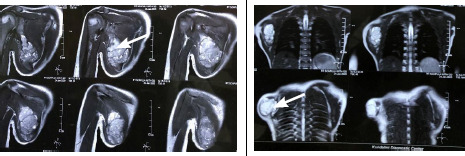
A) An MRI scan showed an ill-defined altered signal intensity lesion measuring 49x35 mm noted at the infraspinatus portion of the body of the scapula, B) heterogeneous enhancement of the lesion on the post-contrast study.
